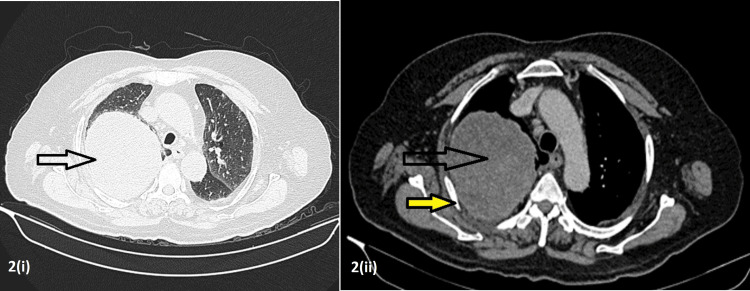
Contrast-enhanced computed tomography (CECT) of the thorax It shows a well-defined heterogeneously enhancing mass in the right upper lobe with areas of necrosis approximately 10.7 x 9.1 x 9 cm (black arrows in both images 2(i) and 2(ii)). Atelectatic changes were also seen in the adjacent lung parenchyma, which were abutting and compressing the right upper lobe and segmental bronchi. Extension of the mass into the chest wall was seen in the posterior aspect with erosions of the third rib (yellow arrow in image 2(ii)).
